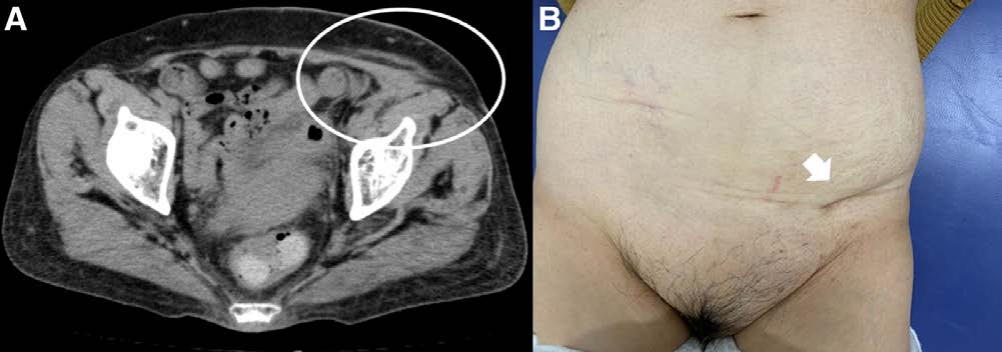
(A) Computed tomography confirmed the disappearance of the abscess in the left inguinal region (circle). (B) Complete epithelialization of the wound on the 60th day after the drainage (arrow).
